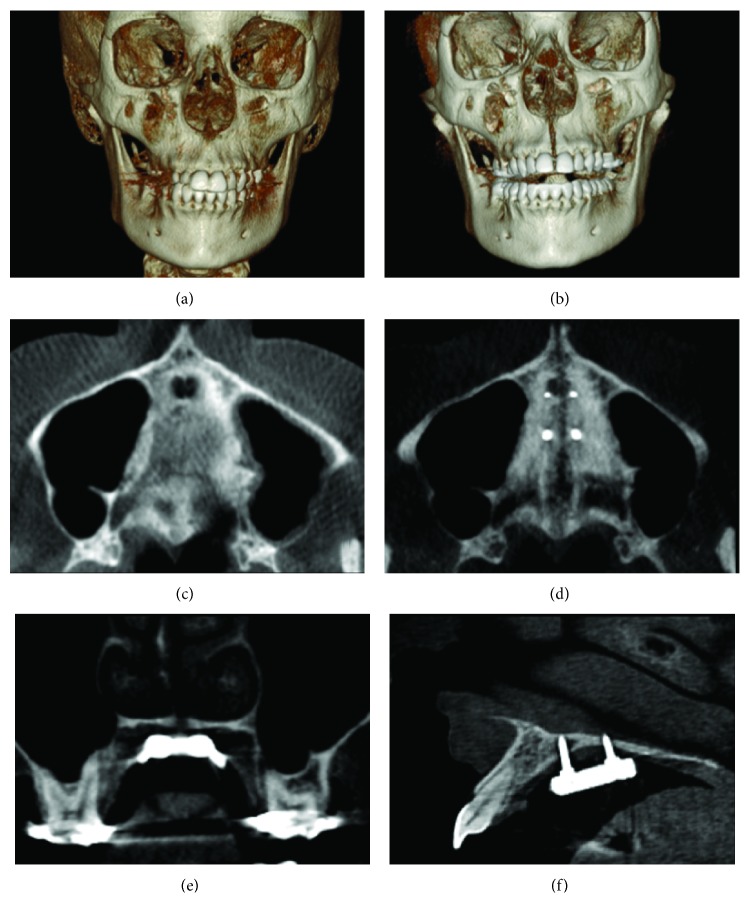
CBCT images after MARPE and corticopuncture procedure: (a, b) 3D reconstructed image in frontal view before and after expansion; (c, d) axial section of the maxilla at the level of anterior and posterior nasal spine before and after expansion; (e) coronal section at the level of the upper first molars showing suture split and molar inclination; and (f) sagittal section showing the bicortical anchorage of the miniscrews.
